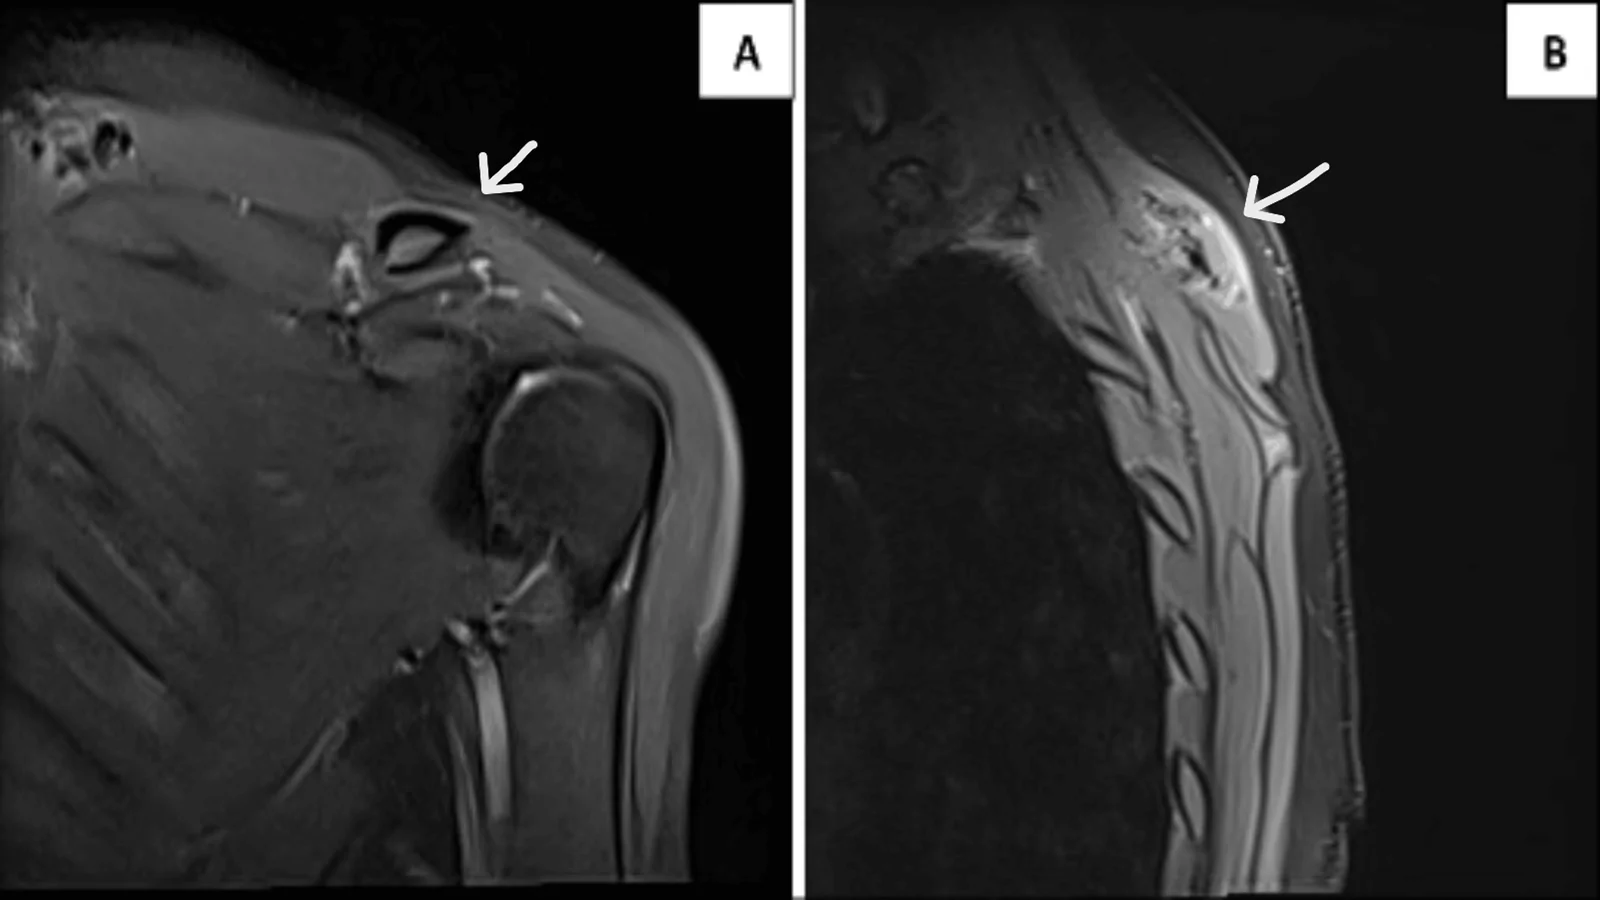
Magnetic resonance imaging of the left trapezius region. (A) Sagittal image showing an ill-defined intramuscular lesion within the left trapezius. (B) Coronal image demonstrating a tangle of serpentine, dilated vessels (flow voids) consistent with a high-flow intramuscular vascular malformation.
